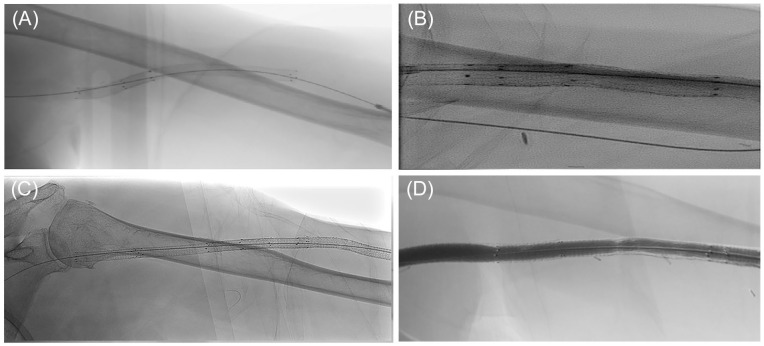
X-ray of covered stents in different patients. (A) After 6 months of cannulation. (B) After approximately 12 months of cannulation. (C) After 18 months of cannulation. (D) An angiographic example of a covered stent after approximately 2 years of cannulation.
